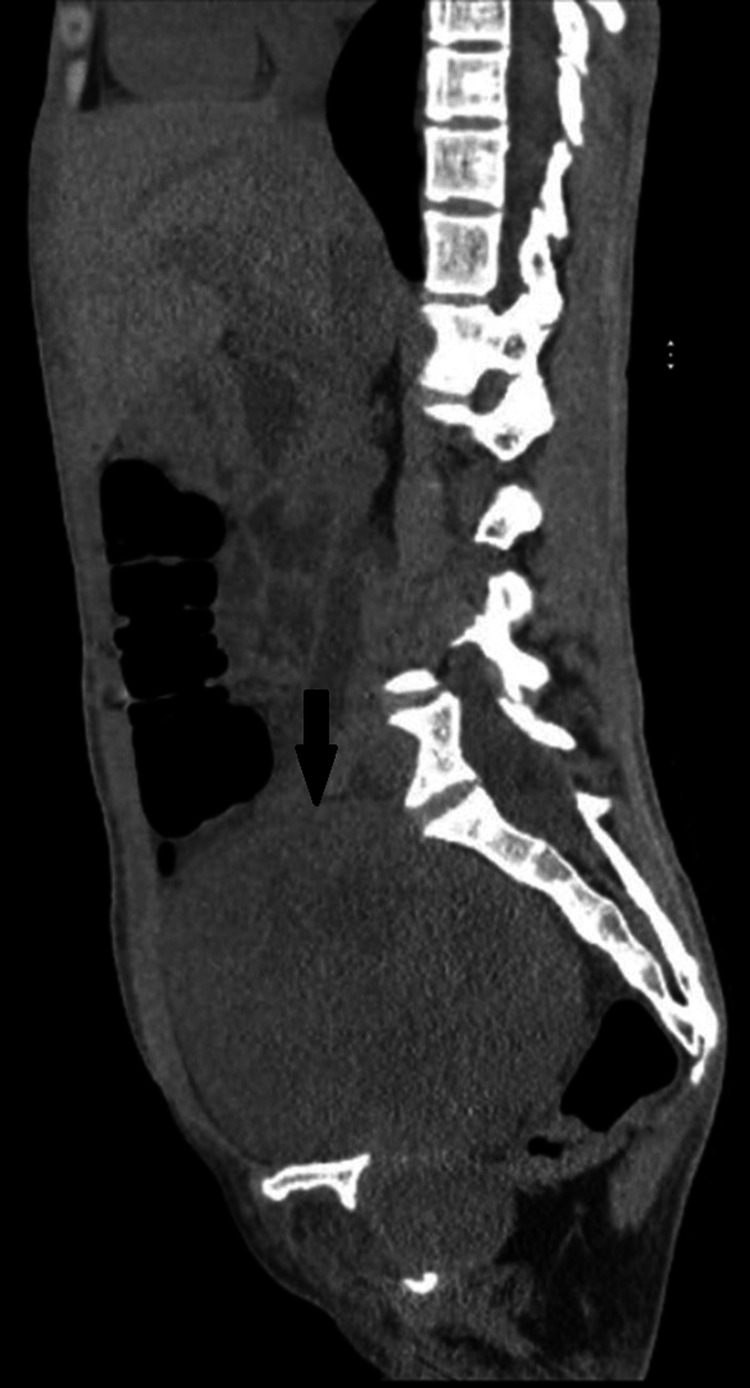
A CT scan of the abdomen and pelvis (sagittal view) The black arrow points to the pelvic mass.
